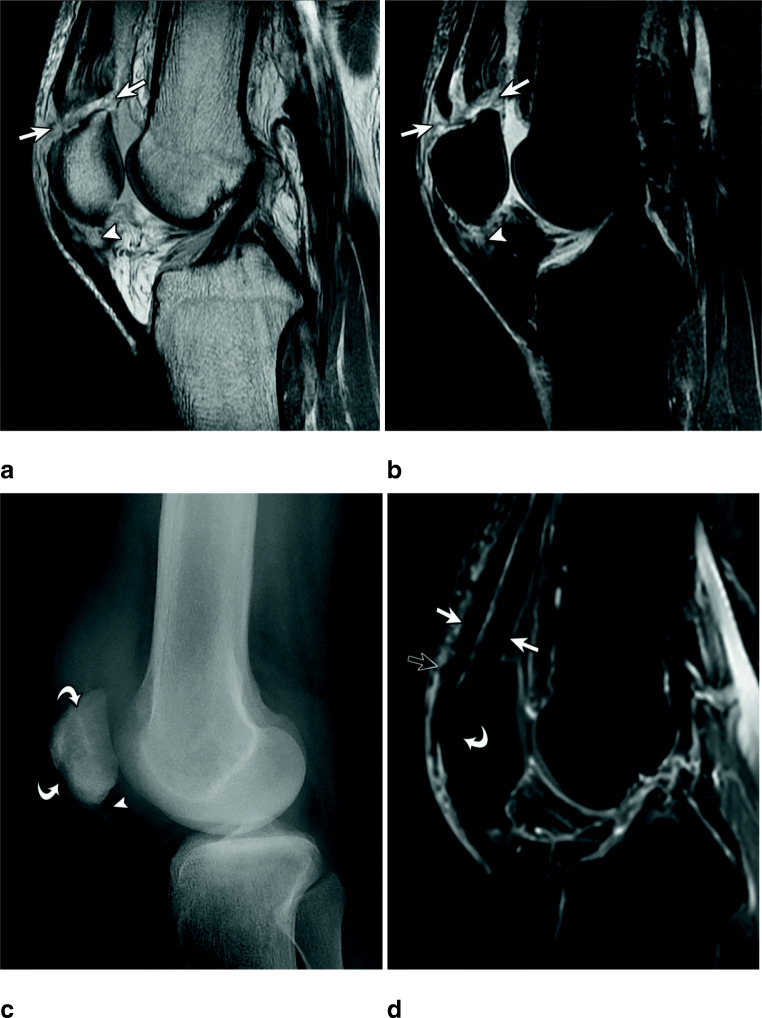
Thirty-three-year-old male with distal quadriceps femoris tear. Intermediate-weighted MR images without (a) and with (b) fat saturation show complete quadriceps tendon rupture at the osseotendinous junction (arrows). Note partial tear of the proximal patellar tendon (arrowhead). The patient was treated surgically with transpatellar bone tunnel tendon fixation (curved arrows) shown on the four weeks postoperative follow-up lateral knee radiograph (c). In addition, an ossification at the site of the proximal patellar tendon tear is seen (arrowhead). Sagittal T1 fat-saturated MR imaging (d) performed 8 years after surgery shows an intact quadriceps tendon (arrows), postsurgical susceptibility artifacts (open arrow), and a completely ossified surgical tunnel (curved arrow)
